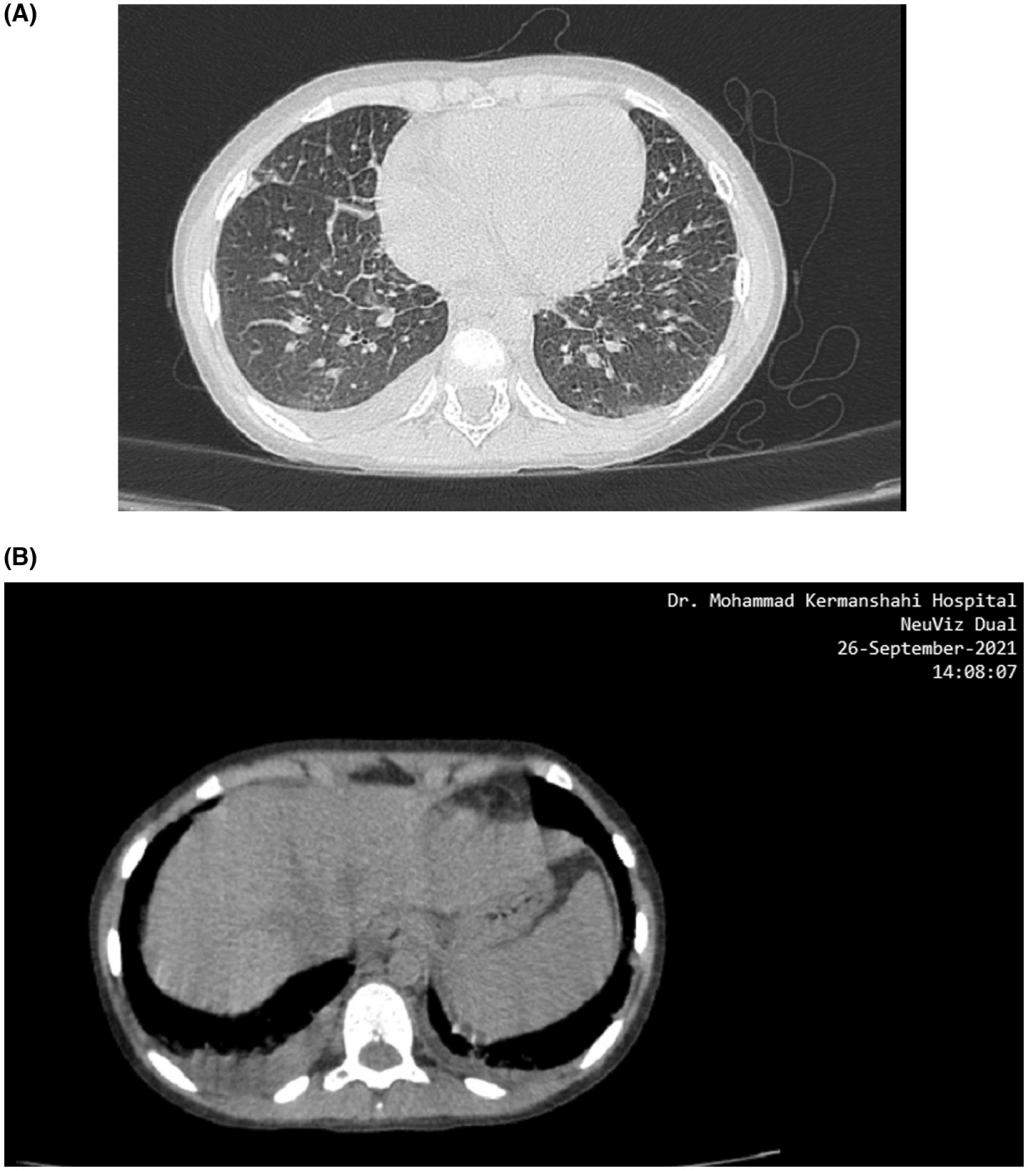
(A, B) Chest CT (computerized tomography) scan showing (A). bilateral pleural effusion (8 mm on right and 4 mm on the left), (B) mediastinal lymphadenopathy (the biggest = 7 mm), and (C) subsegmental atelectasis in the right middle lobe (RML) and right lower lobe (RLL).
